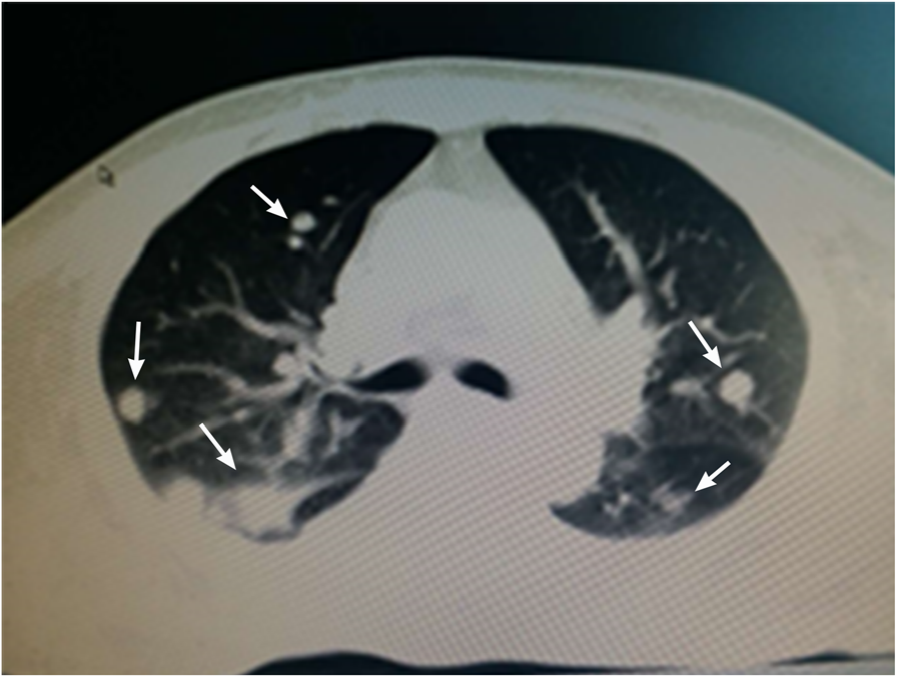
CT image of bilateral lung metastasis of tumours. A CT image was taken cross both lungs at the thoracic level from the subject of case 1. Arrows indicate multiple metastases of tumours to both sides of the lungs
